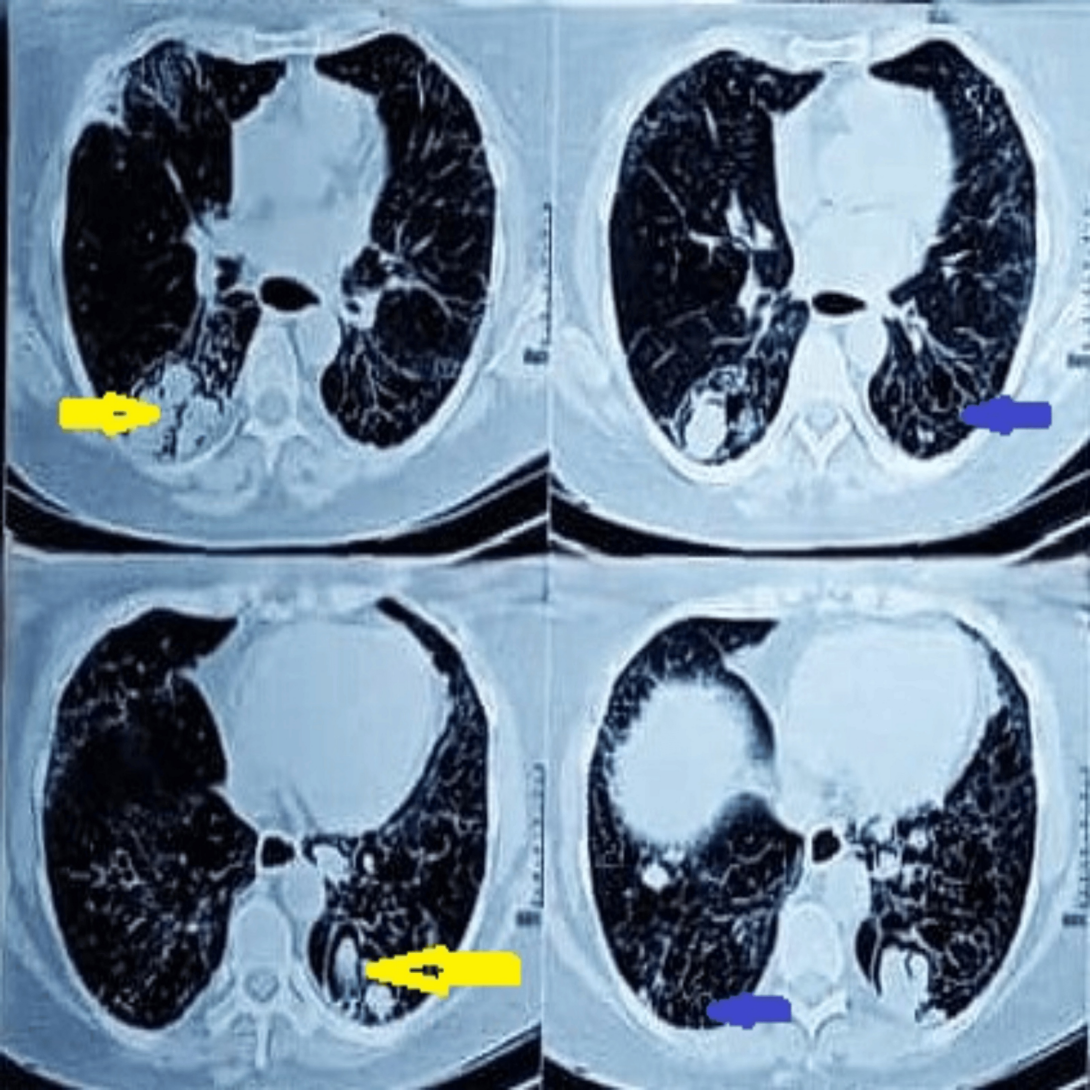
HRCT showing a cavitary lesion with a fungal ball (aspergilloma) on the background of DPLD Non-enhancing soft tissue density lesion (aspergilloma) with a peripheral crescentic margin, with associated air space (yellow arrows on right and left sides) densities noted on both lung fields, and interstitial thickening with honeycombing (blue arrow) are seen in both basal regions. HRCT: high-resolution computed tomography, DPLD: diffuse parenchymal lung disease
